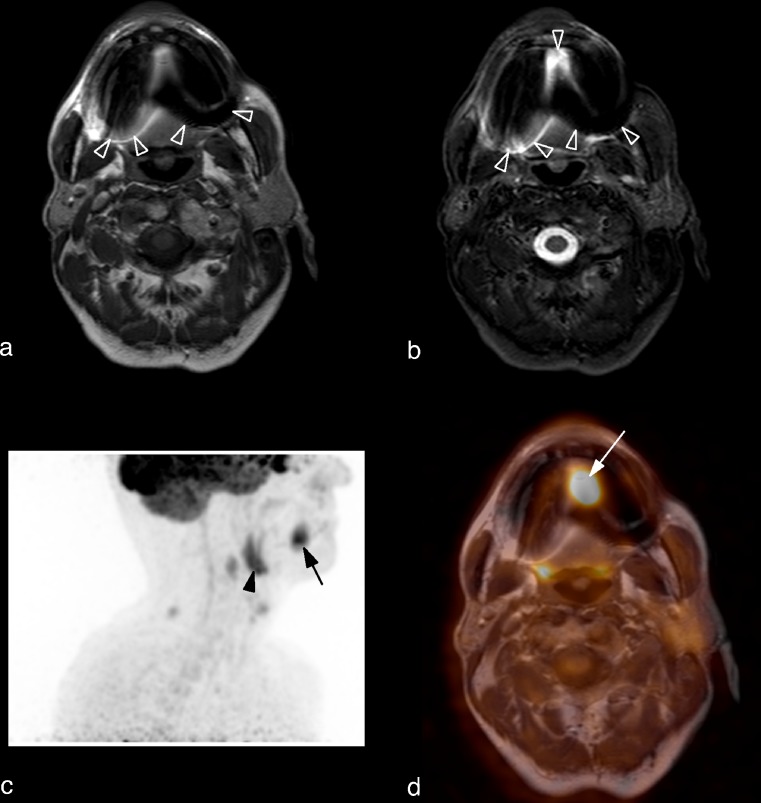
Squamous cell carcinoma of the tongue in a 55-year-old man. a Axial T1-weighted TSE image. The tumour is not detectable due to susceptibility artefacts caused by dental implants (*arrowheads*). b Axial STIR TSE image showing nearly identical susceptibility artefacts (*arrowheads*). c Maximum intensity projection image of the PET dataset showing the tumour (*arrow*) and a lymph node metastasis (*arrowhead*). d Axial fused PET/MR image. The tumour is clearly visible (*arrow*)
